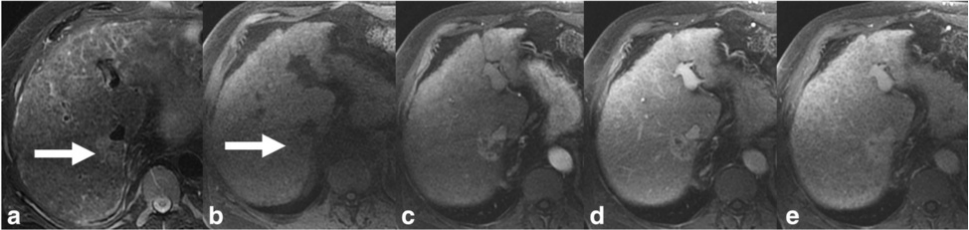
Cirrhosis of liver with iCCA. T2-weighted axial (a), T1-weighted axial (b) and post gadolinium enhanced T1-weighted axial arterial phase (c), portal venous phase (d) and delayed phase (e) images showing iCCA as an iso- to hyperintense lesion (arrow) in posterior right lobe with typical arterial phase rim like enhancement and progressive central enhancement through delayed phase without any washout. The liver parenchyma is heterogeneous and nodular consistent with cirrhosis.
